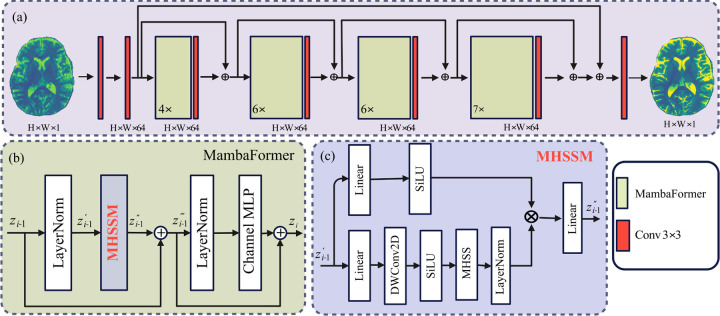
Proposed Vision Mamba framework for MRI super-resolution. (a) Hybrid selective scanning extracts patch sequences. (b) Each MambaFormer block integrates LN, MHSSM, and Channel MLP with residual connections. (c) Multi-head selective state-space modeling runs m parallel scans with adaptive step sizes.
